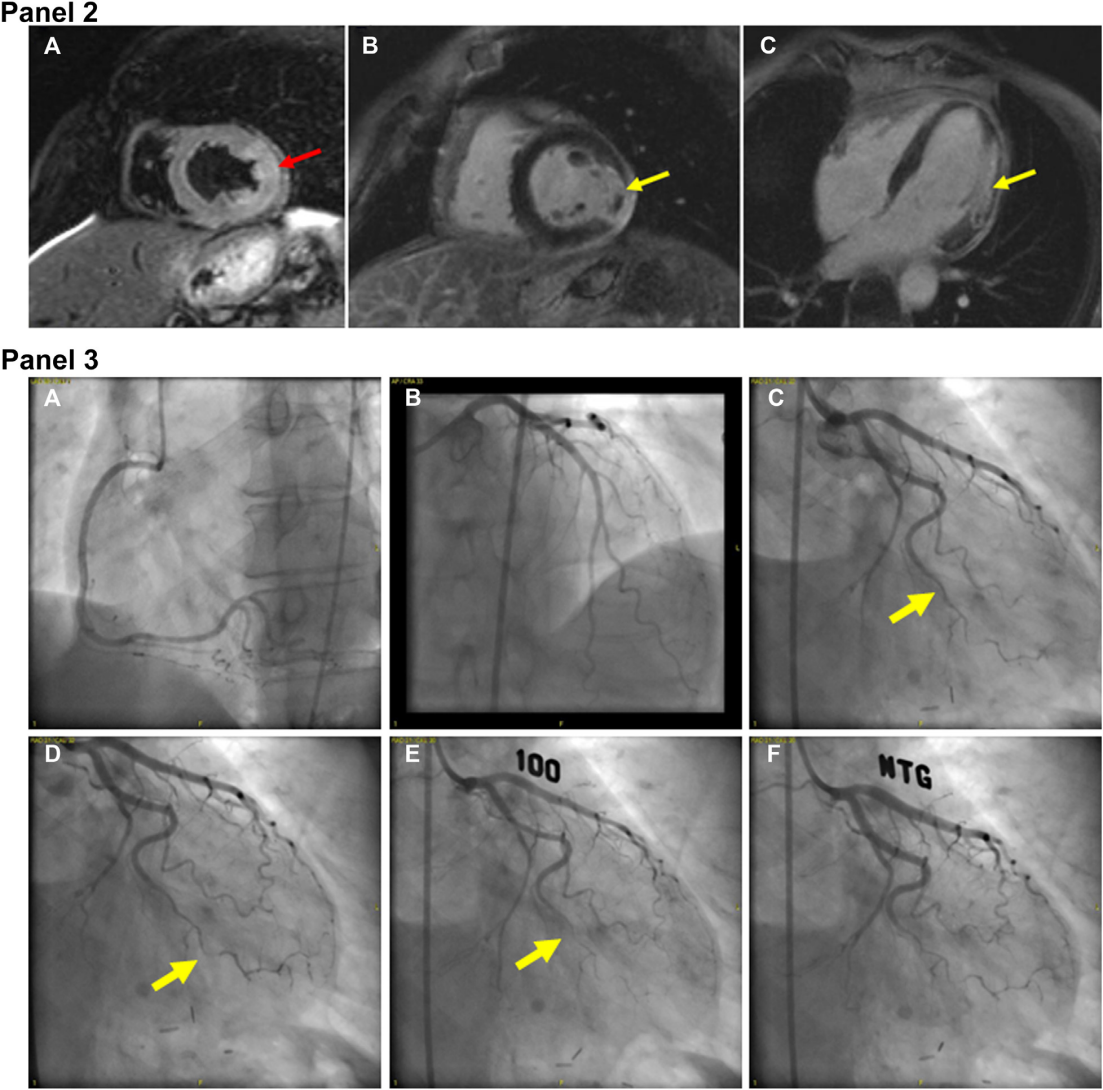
Coronary computed tomography angiogram, cardiac magnetic resonance, and invasive coronary angiogram images of the patient.Panel 1. Representative coronary computed tomography angiogram multiplanar reformatted images representing the left anterior descending (A), left circumflex (B), right (C), and obtuse marginal (D) coronary artery territories. The first obtuse marginal artery is also demonstrated on 3D volume-rendered reconstruction (E). Panel 2. Cardiac magnetic resonance imaging. T2-weighted signal acquisition demonstrating diffuse enhanced T2 signal in the mid lateral wall (A, red arrow), which also extends to the apical lateral segment (not shown). Short-axis (B) and 4-chamber (C), postcontrast acquisition after infusion of gadoterate meglumine demonstrating transmural late gadolinium enhancement in the mid lateral segments with central signal hypointensity (yellow arrow) and adjacent delayed pericardial enhancement. Panel 3. Invasive coronary angiogram, demonstrating no atherosclerotic disease in the right coronary (A), left anterior descending (B), or left circumflex (C) arteries, yet with evidence of dissection (C, arrow) and spasm (D, arrow) of the distal first obtuse marginal (OM1) artery. With 100 μg intracoronary acetylcholine, symptomatic vasospasm of the OM1 (arrow) occurred with 1-mm ST-segment depression (E) and was relieved with intracoronary nitroglycerin (F).
